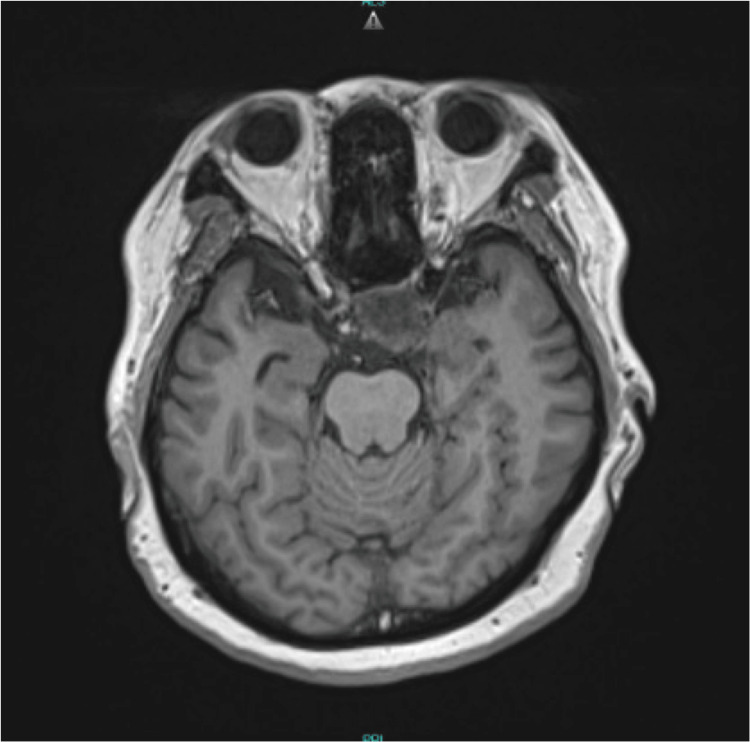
Brain magnetic resonance imaging (MRI) from February 2025. MRI revealed a 2.2 cm pituitary adenoma with significant compression of the optic chiasm.
